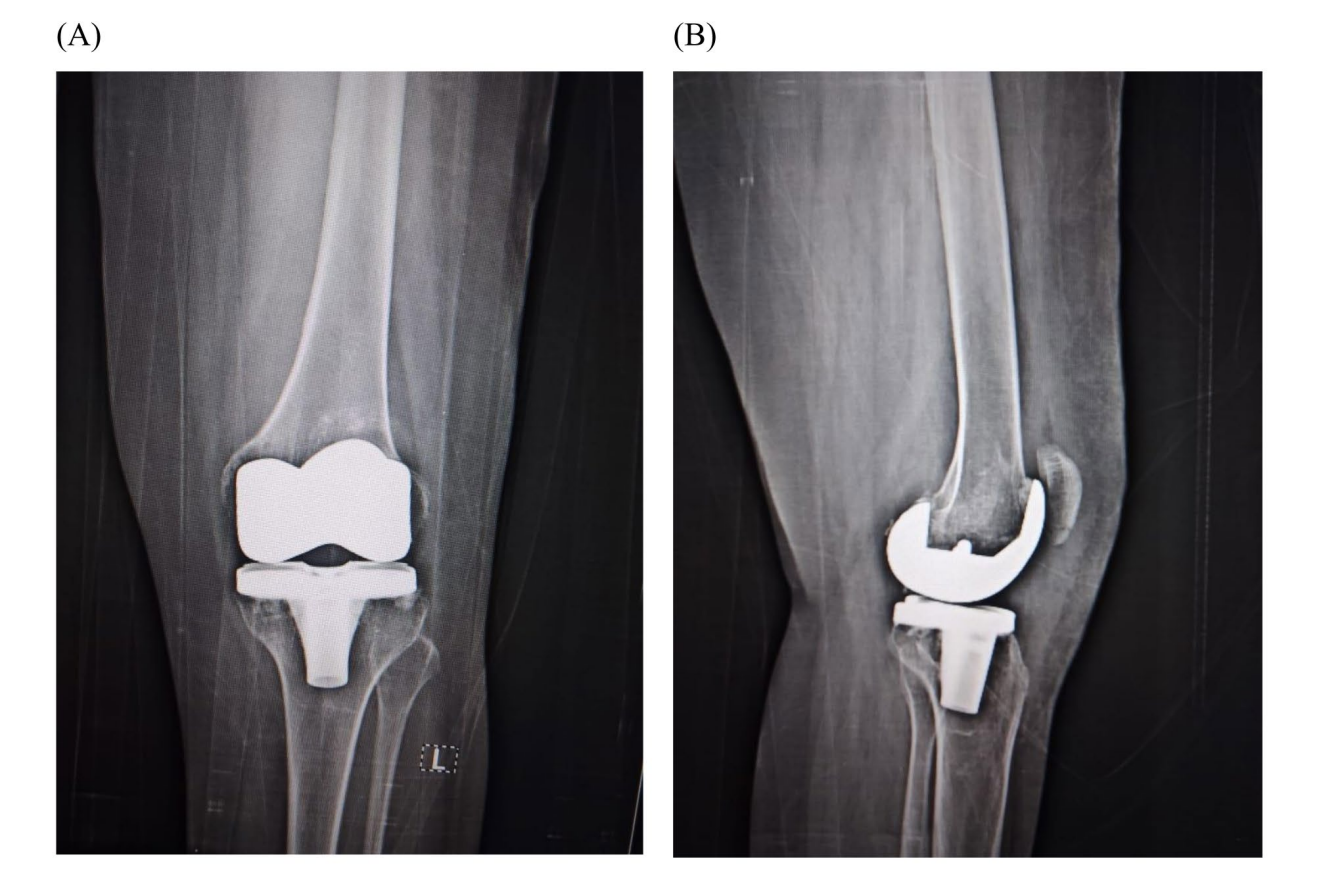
Postoperative antero-posterior (A) and lateral (B) x-rays of the left knee
